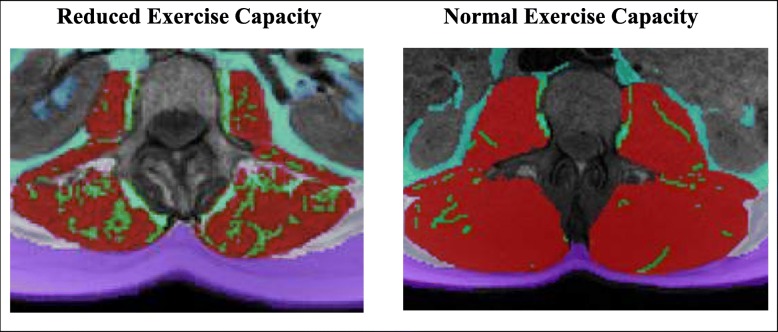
The intermuscular fat of paraspinal muscles, depicted from MRI images of increased IMF (green) relative to SM (red) in a cancer survivor with reduced exercise capacity (left) and a control participant with normal exercise capacity (right)
